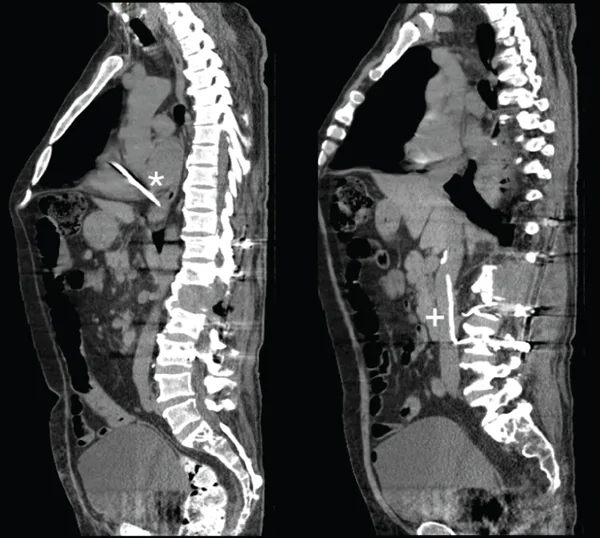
Sagittal computed tomography scan showing an infiltrative extradural lesion affecting the vertebral bodies from T11 to L2, consistent with metastatic spinal cord compression. Additionally, two hyperdense linear structures consistent with embolized cement are seen: one perforating the right ventricle (∗) and another ascending through the inferior vena cava from the lumbar venous plexus (+).
